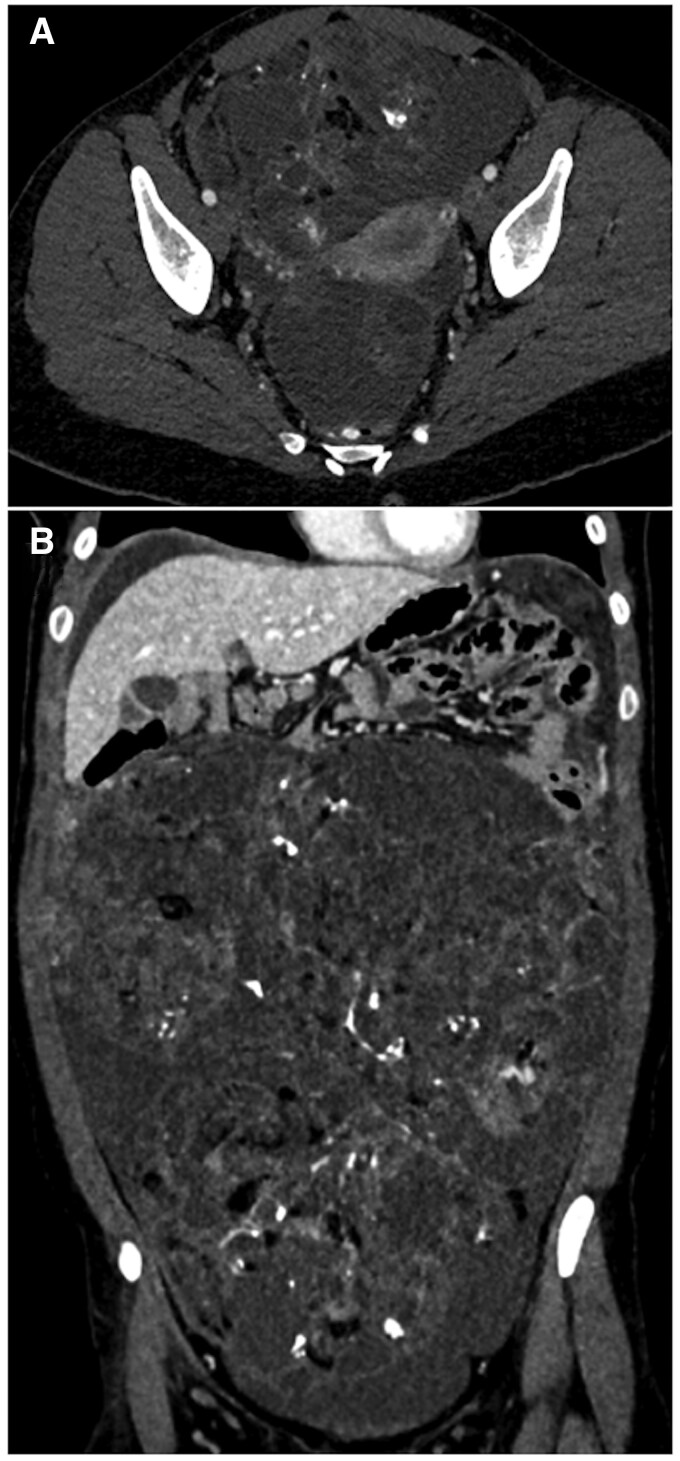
Dysgerminoma. CT images of a 19-year-old girl showing a large multilocular lesion with enhancing fibrovascular septa of different thicknesses, in keeping with ovarian dysgerminoma. Note the absence of peritoneal or nodal involvement despite the lesion size.
